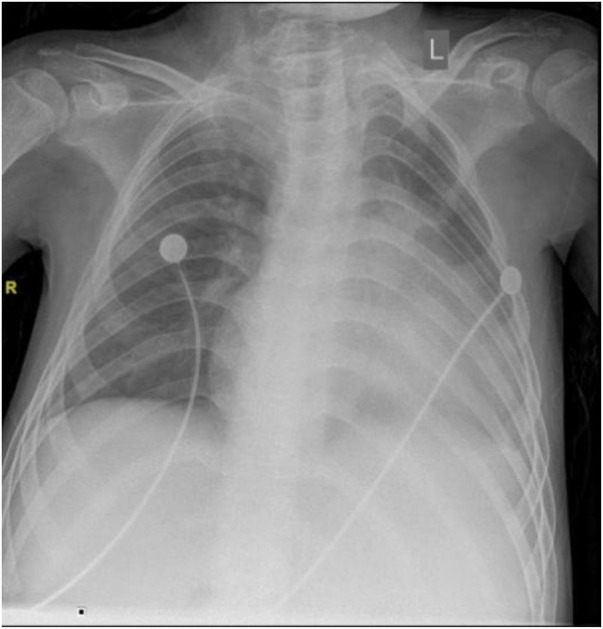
Chest x-ray on September 23, 2021.
